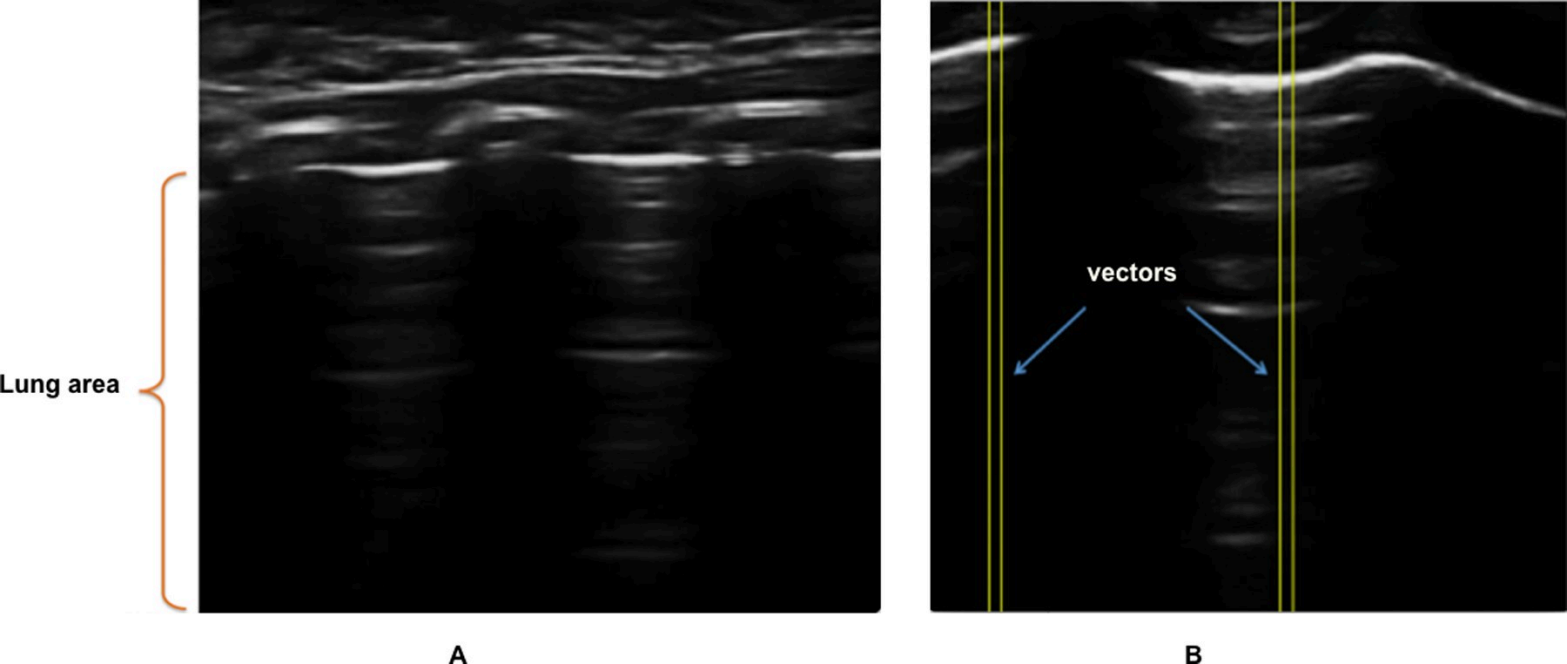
(A) Ultrasound of the lung, (B) Segmentation of (A) in vectors.
